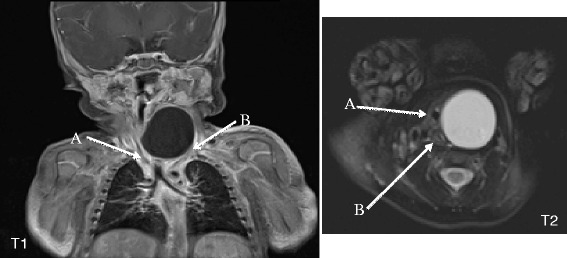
Cervical thoracic MRI. Coronal T1-weighted sequence showing a voluminous left laterocervical mass displacing the trachea (a) and carotid-jugular axis (b). Axial T2-weighted sequence showing tracheal compression (a) and esophageal compression (b) by the cyst
